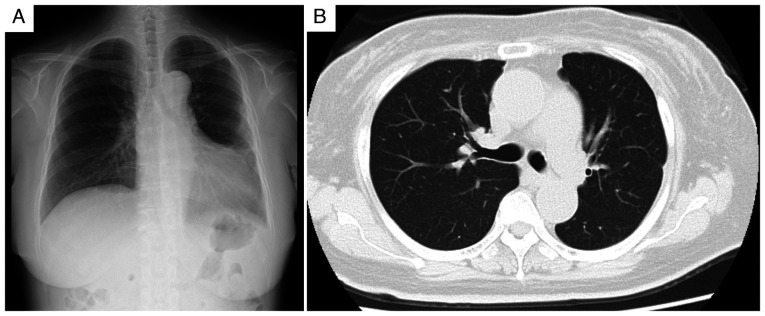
Follow-up radiological findings in May and June 2025, demonstrating sustained treatment response. (A) Chest radiograph showing sustained resolution of pleural effusion. (B) Chest computed tomography confirming the complete resolution of effusion and no new parenchymal abnormalities.
